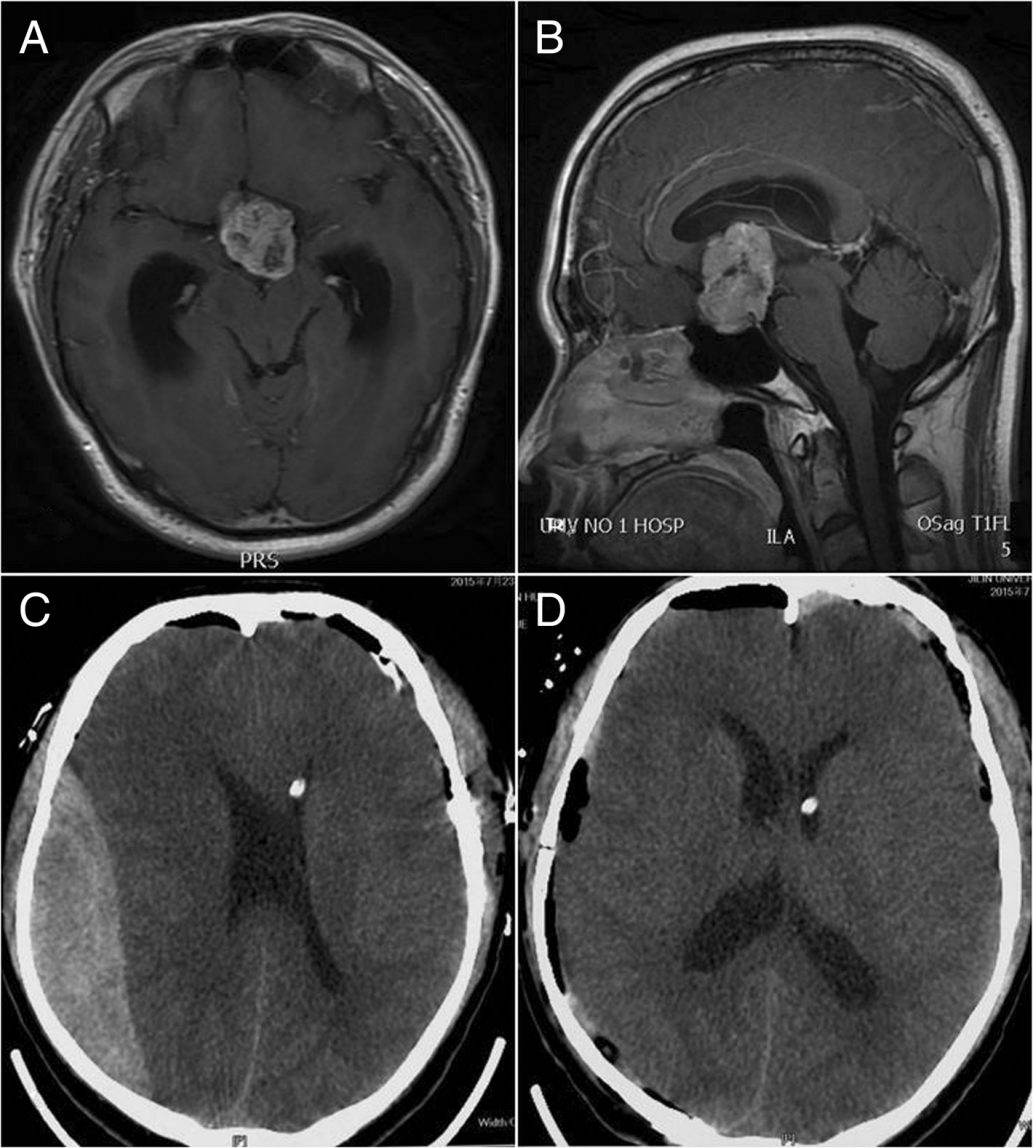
Contralateral remote hemorrhage in case 9. a, b Preoperative-enhanced MRI revealed a sellar tumor, with ventricular dilatation. c CT showed a contralateral epidural hematoma in the surgical field after the tumor resection, with an intraventricular drainage tube. d CT showed that the hematoma had been cleared
